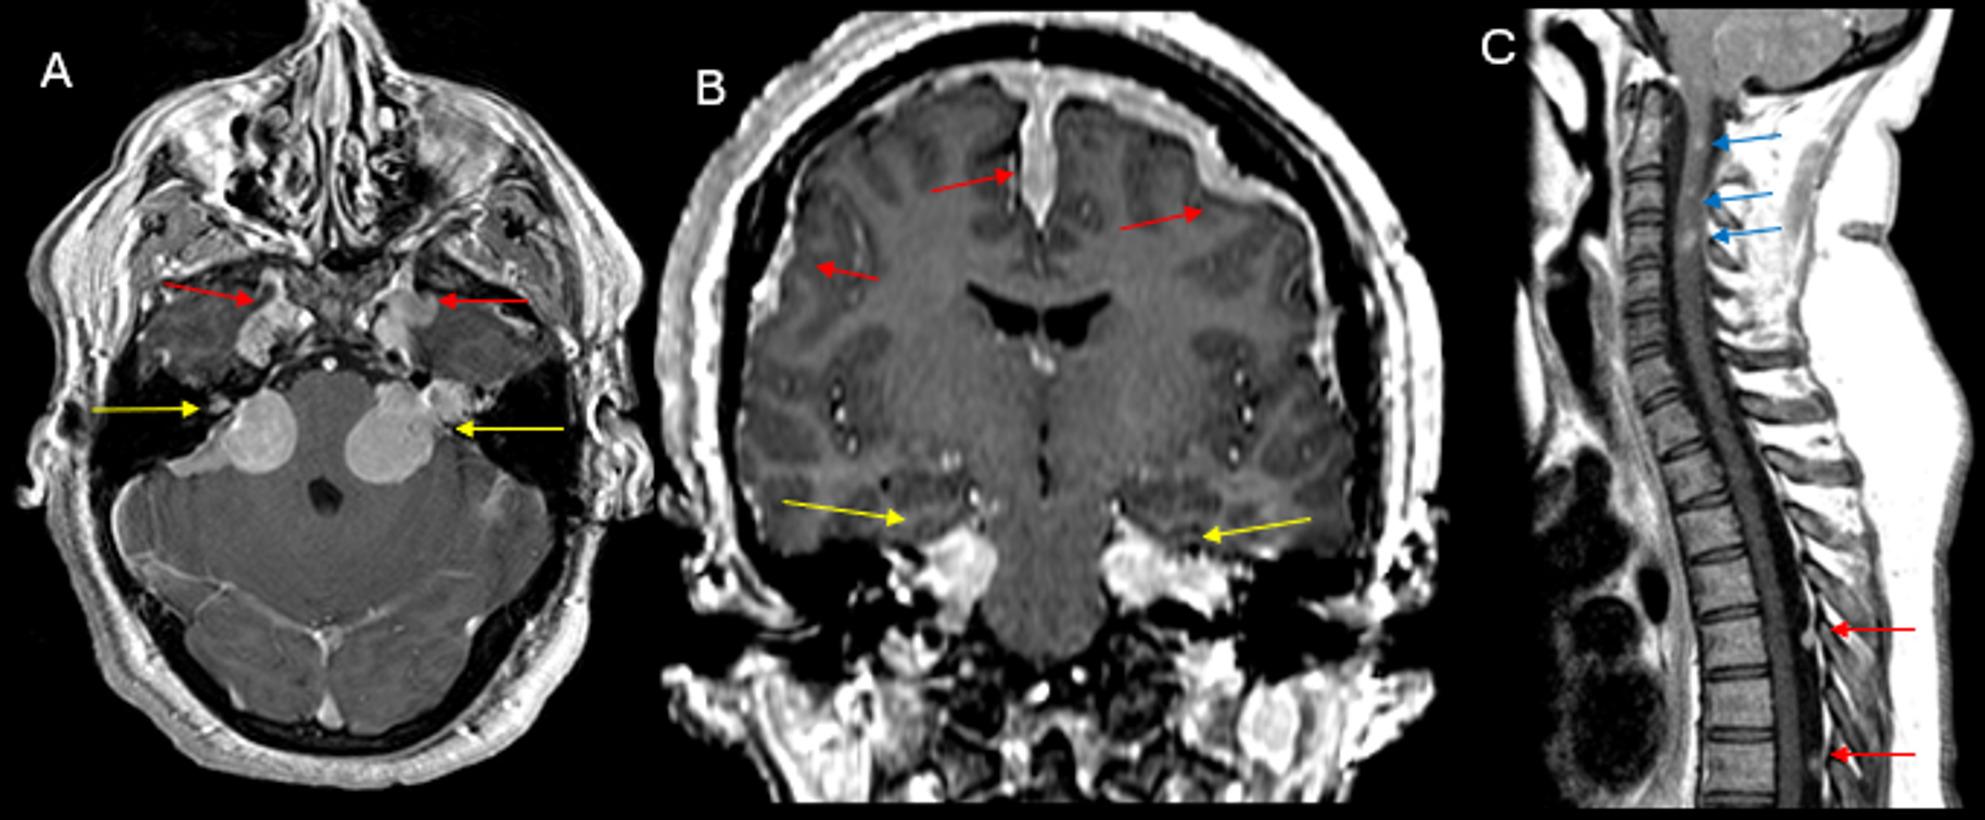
(A) Post-contrast axial T1-weighted and (B) coronal T1-weighted images demonstrate enhancing bilateral vestibular schwannomas (yellow arrows) and multifocal meningiomas along the cerebral convexity, falx, and bilateral mesial temporal regions (red arrows) in a patient with NF2. (C) Post-contrast sagittal T1-weighted image of the cervical and upper thoracic spine shows a few enhancing intramedullary lesions in the cervical cord, suggestive of ependymomas (blue arrows). Additionally, dural based intraspinal extramedullary lesions in the dorsal mid thoracic spine are also present, compatible with meningiomas (red arrows)
